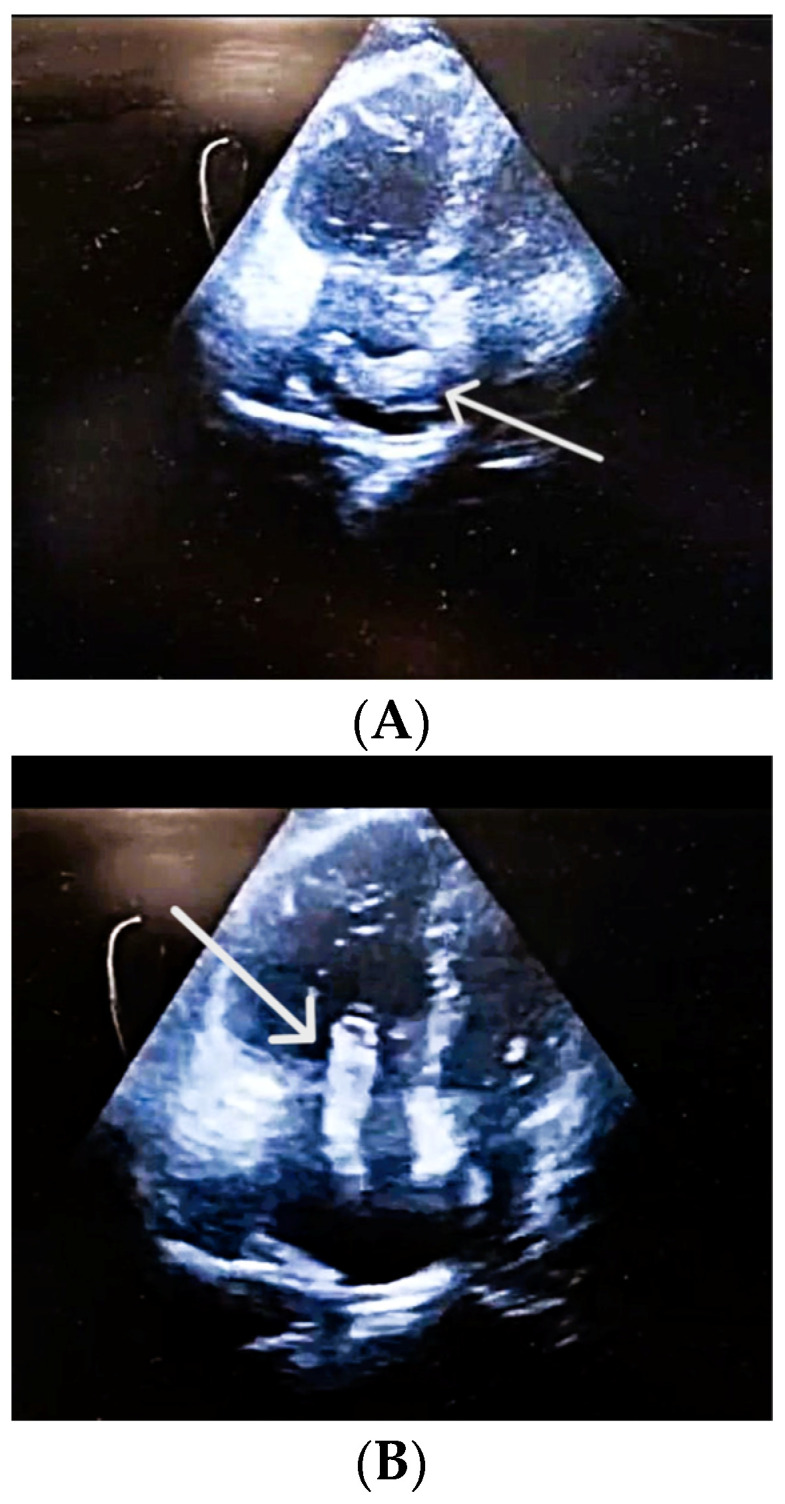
(A) POCUS-TTE performed pre-thrombolysis, showing a thrombus in transit. Apical four-chamber view demonstrating a large, mobile thrombus (in a horizontal position) within the right atrium (white arrow). (B) POCUS-TTE performed pre-thrombolysis, showing a thrombus in transit. Apical four-chamber view showing a large, serpentine, free-floating thrombus (white arrow) within the right atrium, seen prolapsing toward the tricuspid valve. Right ventricular cavity is larger than the left ventricular cavity RV/LV > 1.
